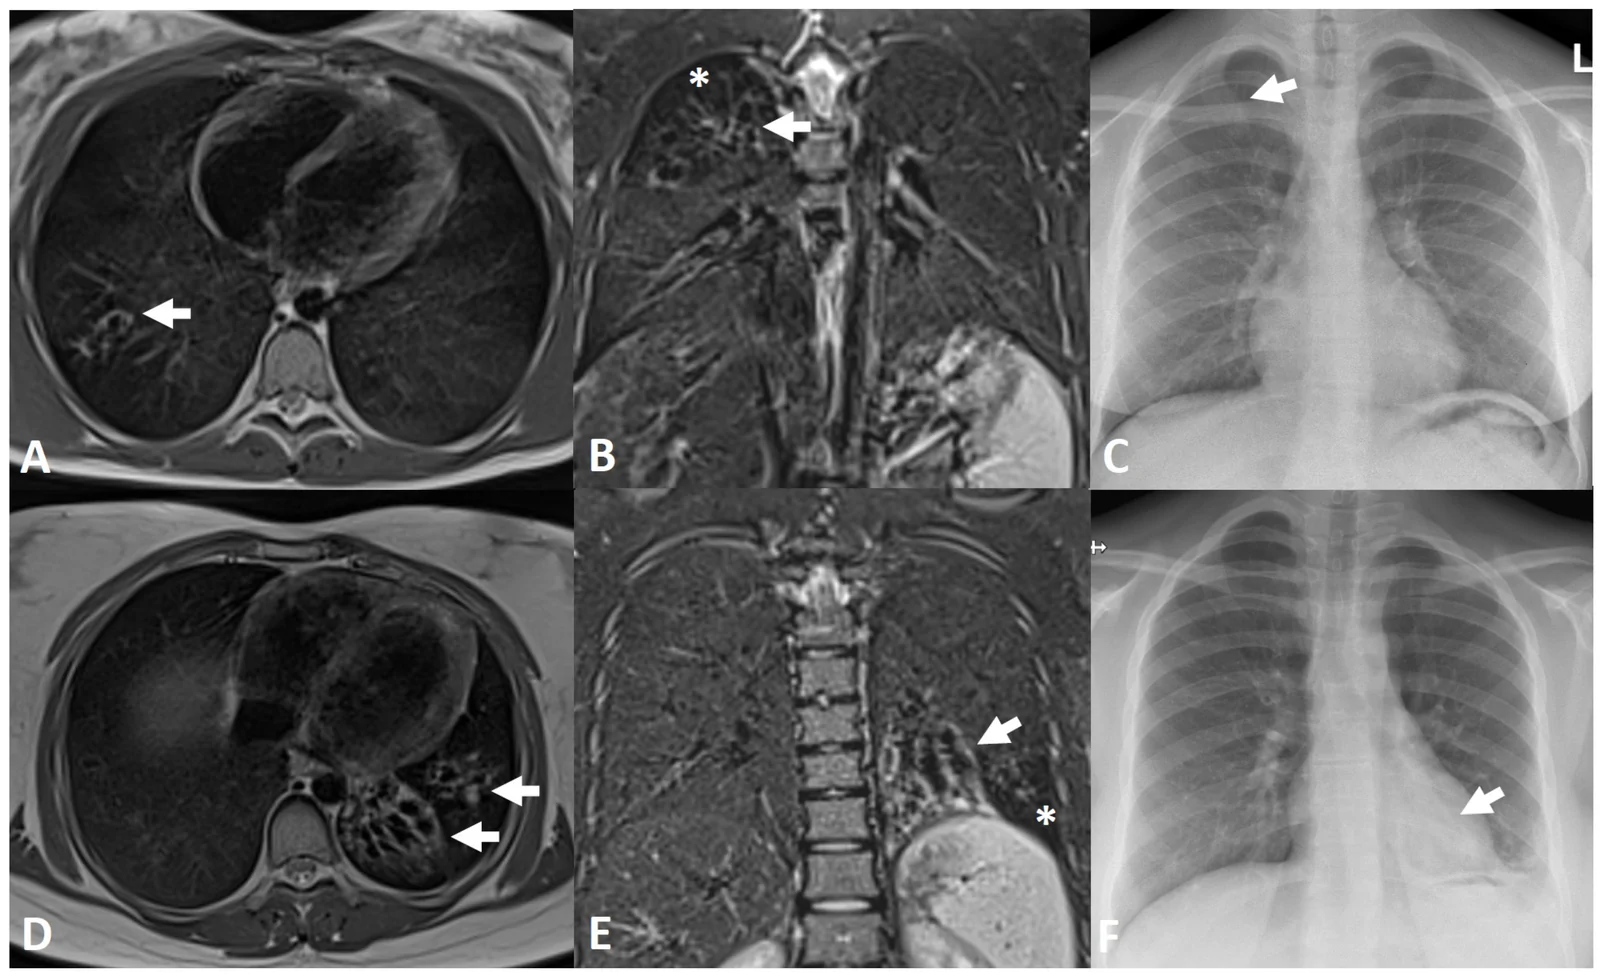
(A,B): MRI of the lung at 0.55 T in a female 14-year-old patient with CF presenting with bronchiectasis and air trapping in the right upper lobe (arrow), as well as air trapping (*) in the lingula. (A): Transversal proton-density-weighted sequence of the lung. (B): Coronal T2-weighted fat-suppressed sequence of the lung. (C): Corresponding chest radiography in posterior–anterior view revealing subtle or barely discernible pulmonary abnormalities, such as bronchiectasis in the right upper zone (arrow). (D,E): MRI of the lung at 0.55 T in a female 17-year-old patient with CF presenting with bronchiectasis (arrow) in the left lower lobe and air trapping (*) in the lingula. (D): Transversal proton-density-weighted sequence of the lung. (E): Coronal T2-weighted fat-suppressed sequence of the lung. (F): Corresponding chest radiography in posterior–anterior view not revealing bronchiectasis in the left lower lobe, as it is obscured by the cardiac silhouette (arrow). Blunted left lateral recess. L, left side marker on the radiograph.
